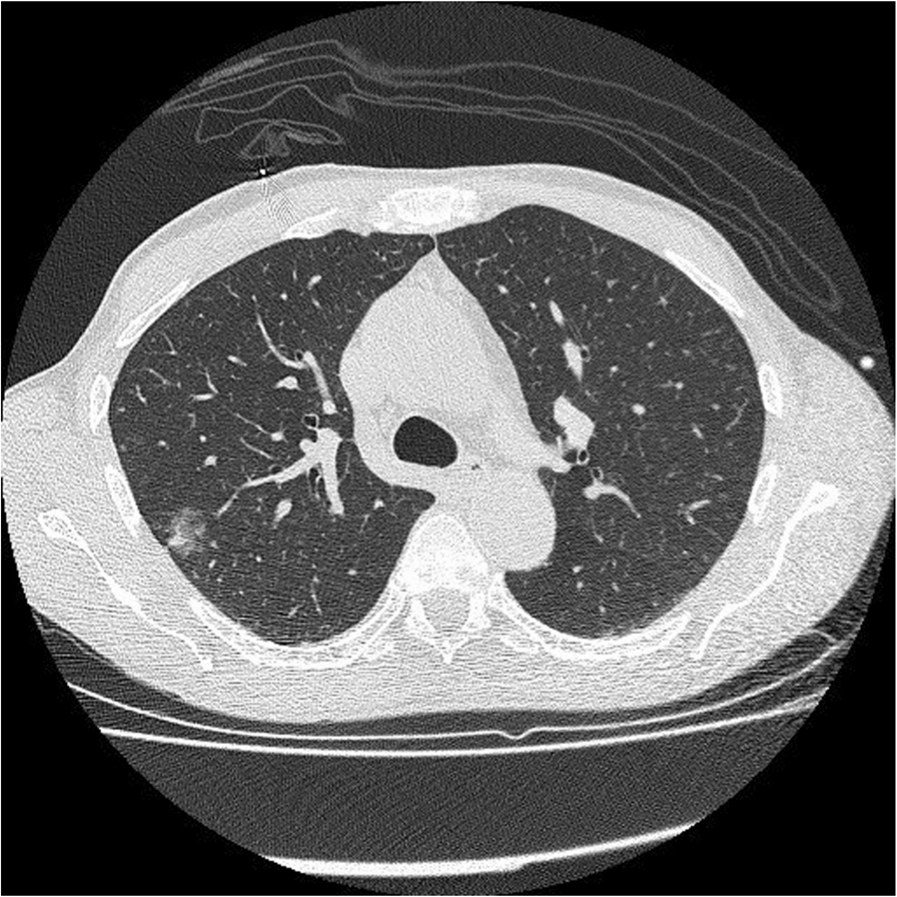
MIA on CT. A 20-mm part-solid nodule, predominantly ground glass, in the right upper lobe
